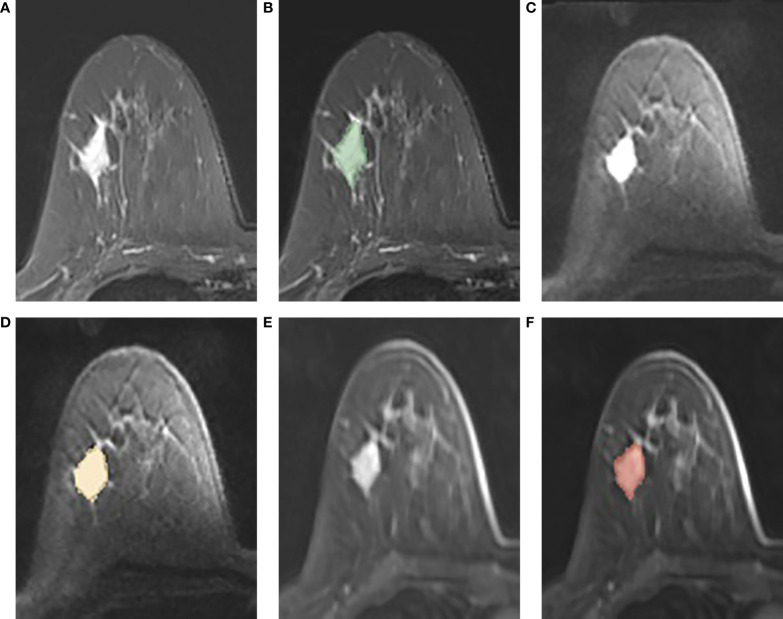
(A-F): An example of manual segmentation in breast cancer. (A, B): tumor area (green in fat suppression T2WI image); (C, D): tumor area (orange in DWI image, b = 1,000 s/mm^2^); (E, F): tumor area (red in DCE-MR image).
